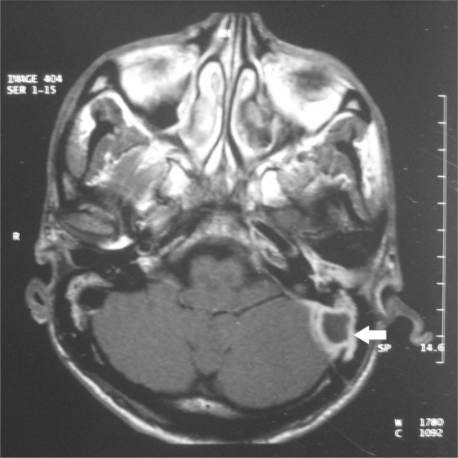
MRI scan showing (arrow) post contrast enhancement of the sinus wall on the left side (with permission from Indian journal of Otolaryngology & head and neck surgery).
